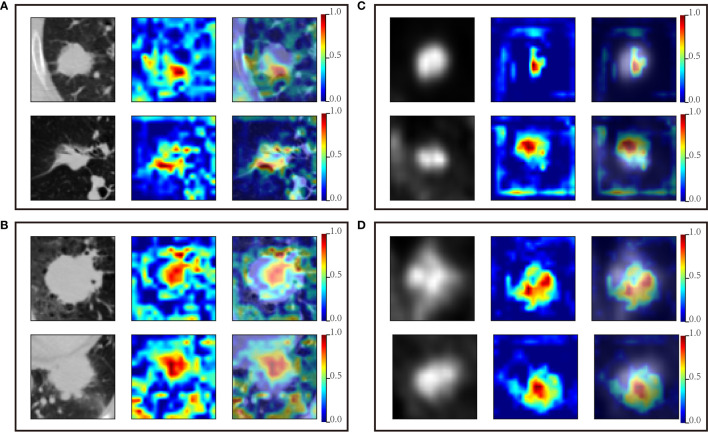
Suspicious areas generated by SE_CT_ and SE_PET_. The first column is the original PET or CT image; the second column is the attention map for classifying EGFR mutation status; the third column is the image fusing original image and the attention map. (A) CT images predicted as EGFR mutation by the SE_CT._
